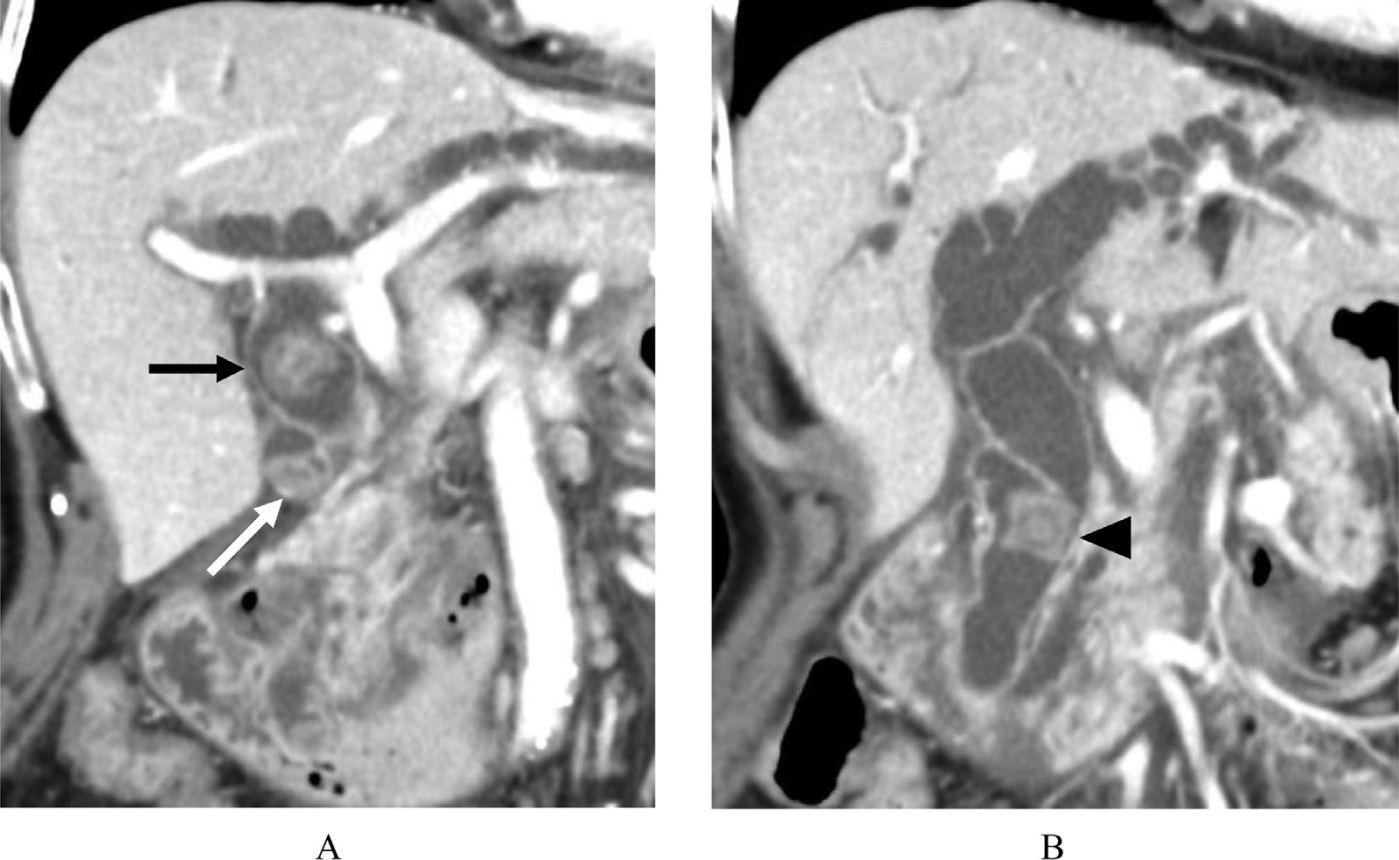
(A) Coronal image of abdominal contrast-enhanced CT shows common hepatic duct stone (black arrow) and cystic duct stone (white arrow). (B) Another coronal image of abdominal contrast-enhanced CT shows common bile duct stone (arrowhead).
